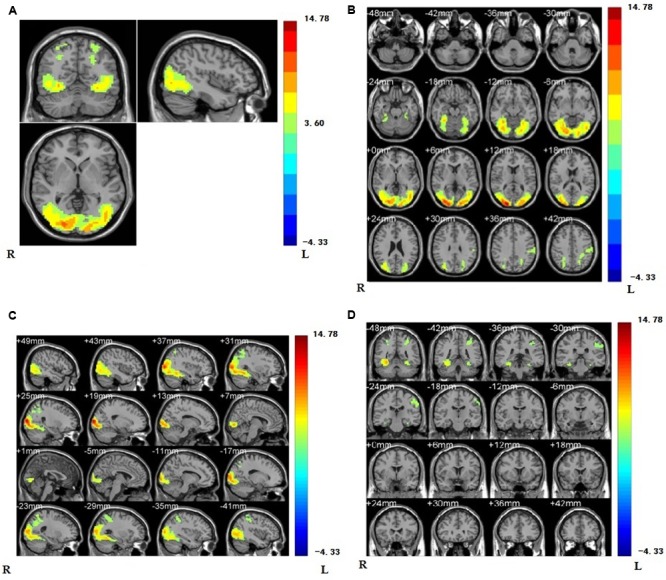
(A) Regions showing higher activation are the regions of supramarginal gyrus, lateral middle occipital gyrus, lateral fusiform gyrus, right inferior occipital gyrus, inferior parietal gyrus, middle temporal gyrus, and visual related regions such as V2 compared with non-painful stimuli (*p* < 0.001, Alphasim corrected; *k* > 1361). We have separately MR imaging at the position of oblique-axial (B) plane, oblique coronal; (C) and sagittalia; (D) in regions activated.
